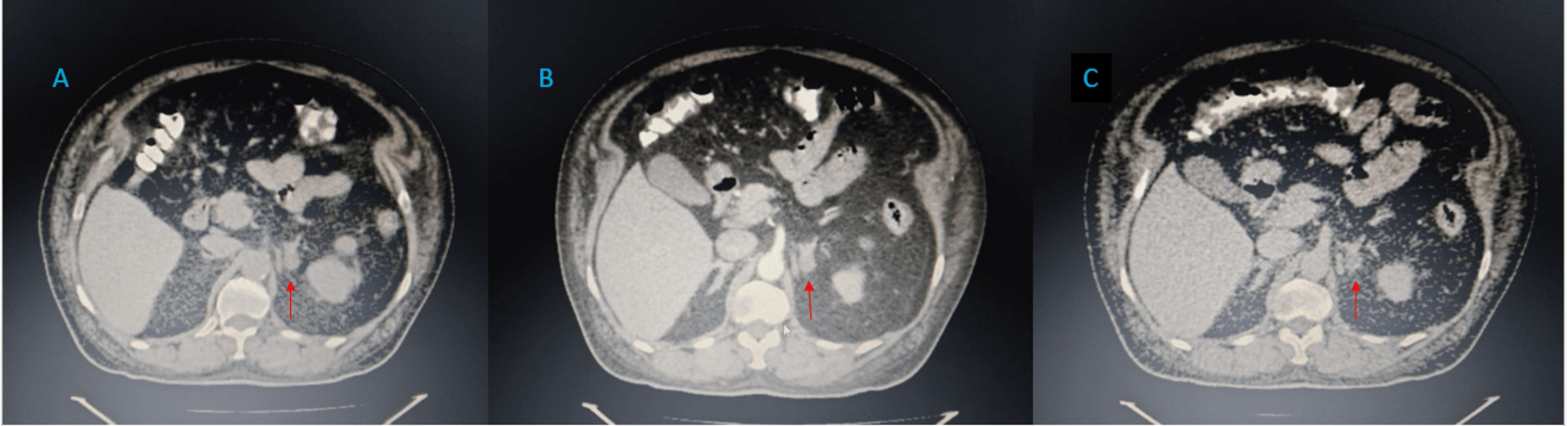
Computed tomography of the adrenal glands demonstrating a left adrenal lesion (A) Non-contrast image showing a small left adrenal lesion with low attenuation (arrow). (B) Arterial phase image demonstrating no significant enhancement (arrow). (C) Venous phase image confirming a non-enhancing lesion (arrow), consistent with a benign adrenal lesion.
